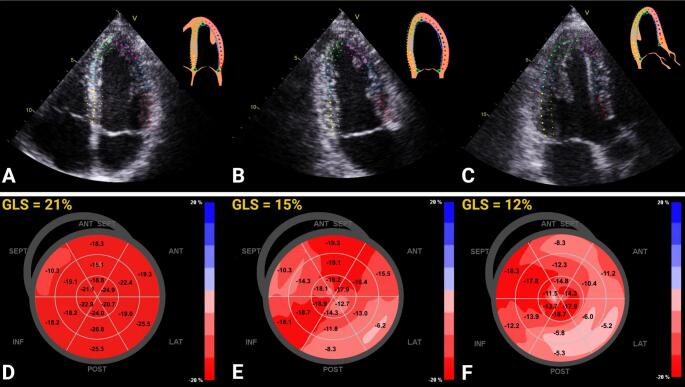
Speckle tracking echocardiography. Panels A–C: Speckle tracking analysis in different echocardiographic views. Panel D: Normal global longitudinal strain (GLS). Panel E: Mildly reduced GLS in a patient with heart failure with preserved ejection fraction (HFpEF). Panel F: Reduced GLS in a patient with heart failure with reduced ejection fraction (HFrEF)
